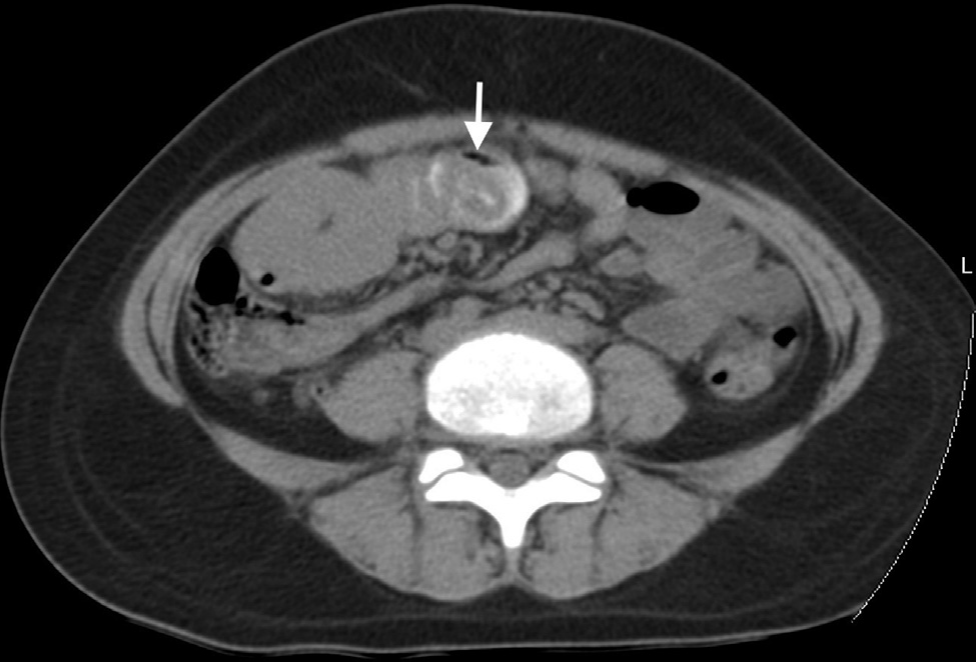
Axial oral contrast-enhanced computed tomographic scan of abdomen demonstrating the telescoping of the jejunal loops suggestive of jejuno-jejunal intussusception (white arrow) with thickening of adjacent jejunal loops.
